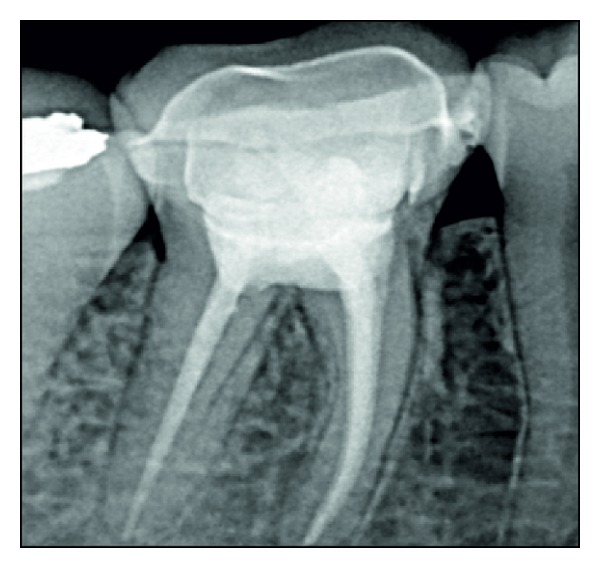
Radiographic view, postcementation. The supragingival finish line is clearly visible.
